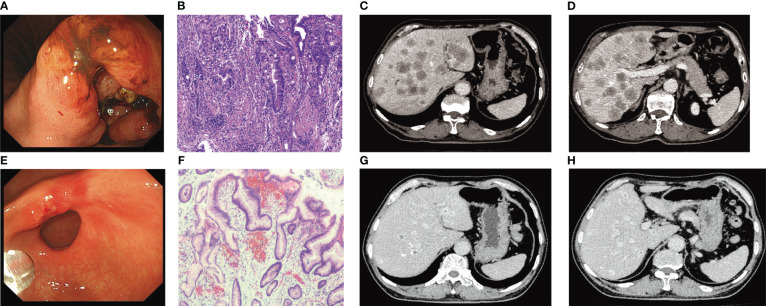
(A) Gastroscopy before treatment. (B) Pathological findings of gastroscopic biopsy (HE×200) (suggesting moderately differentiated adenocarcinoma). (C, D) Abdominal CT before treatment. (E) Results of gastroscopy examination after 6 cycles of treatment (disappearance of lesions before treatment). (F) Pathological examination results after 6 cycles of treatment (HE×200). (G, H) Evaluation of Partial response (PR) after 6 cycles of trastuzumab combined with HAIC treatment.
